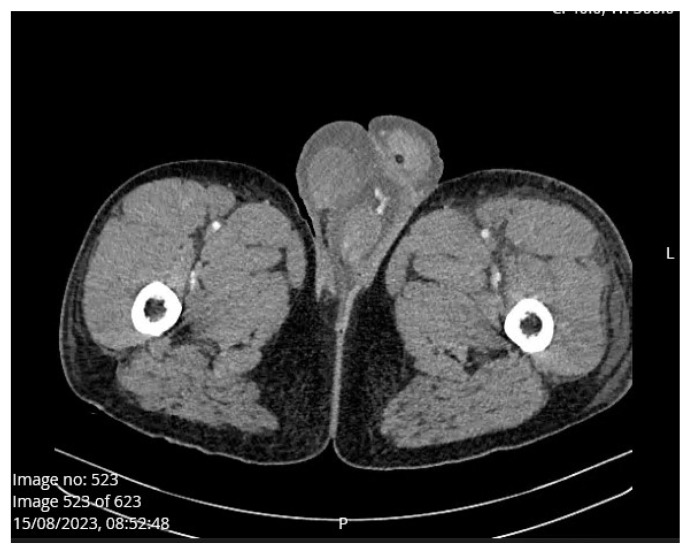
Axial contrast CT scan showed dilated scrotal veins of the pampiniform venous plexus with the indicated caliber of the testicular veins on both sides from the scrotum to the level of the inguinal canal—a progression compared to the earlier finding. There was no sign of acute compartment syndrome. The amount of free fluid in the scrotum and subcutaneous tissue of the penis was also progressing—edema. The testicles, spongy and cavernous bodies of the penis are properly imbibed post-contrast. Bilateral inguinal subcutis edema.
